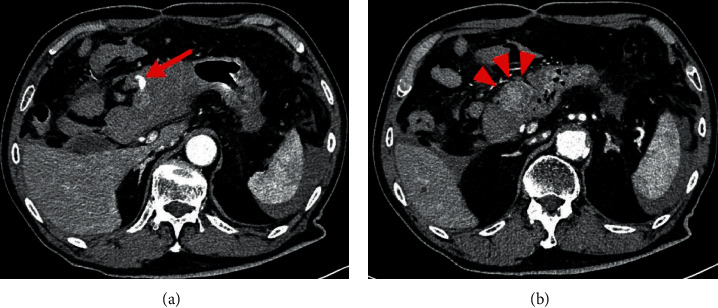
Contrast-enhanced abdominal computed tomography showed contrast leakage ((a), arrow) into the peritoneal cavity from a hypervascular mass ((b), arrowheads, 30 mm) on the gastric antrum's anterior wall.
